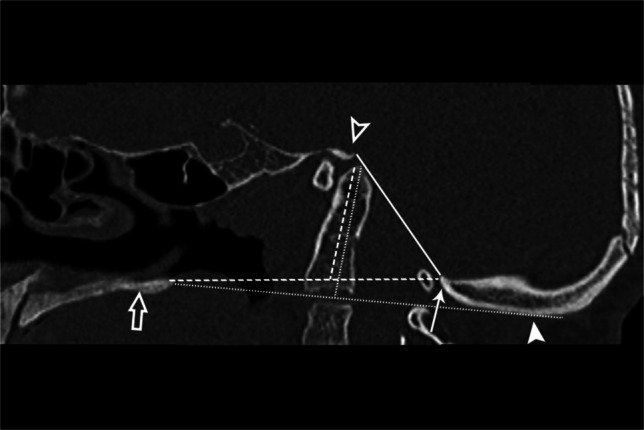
Sagittal CT of the skull base in a 17-year-old female with clear platybasia and basilar invagination. Note that a strict interpretation of the McRae line (*solid line*), drawn from the basion (*hollow arrowhead*) to the opisthion (*arrow*), would not diagnose basilar invagination as the dens does not cross the line. However, the Chamberlain line (*horizontal dashed line*), drawn from the hard palate (*hollow arrow*) to the opisthion, does diagnose basilar invagination as the dens (*vertical dashed line*) extends more than 3 mm above. The McGregor line (*horizontal dotted*
